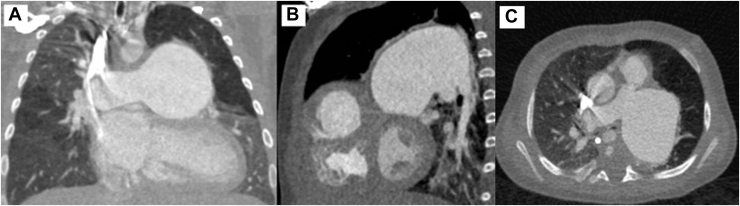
Preoperative computed tomography demonstrates aneurysmal dilatation of both branches of the PA, with severe narrowing of the right and left bronchi.
